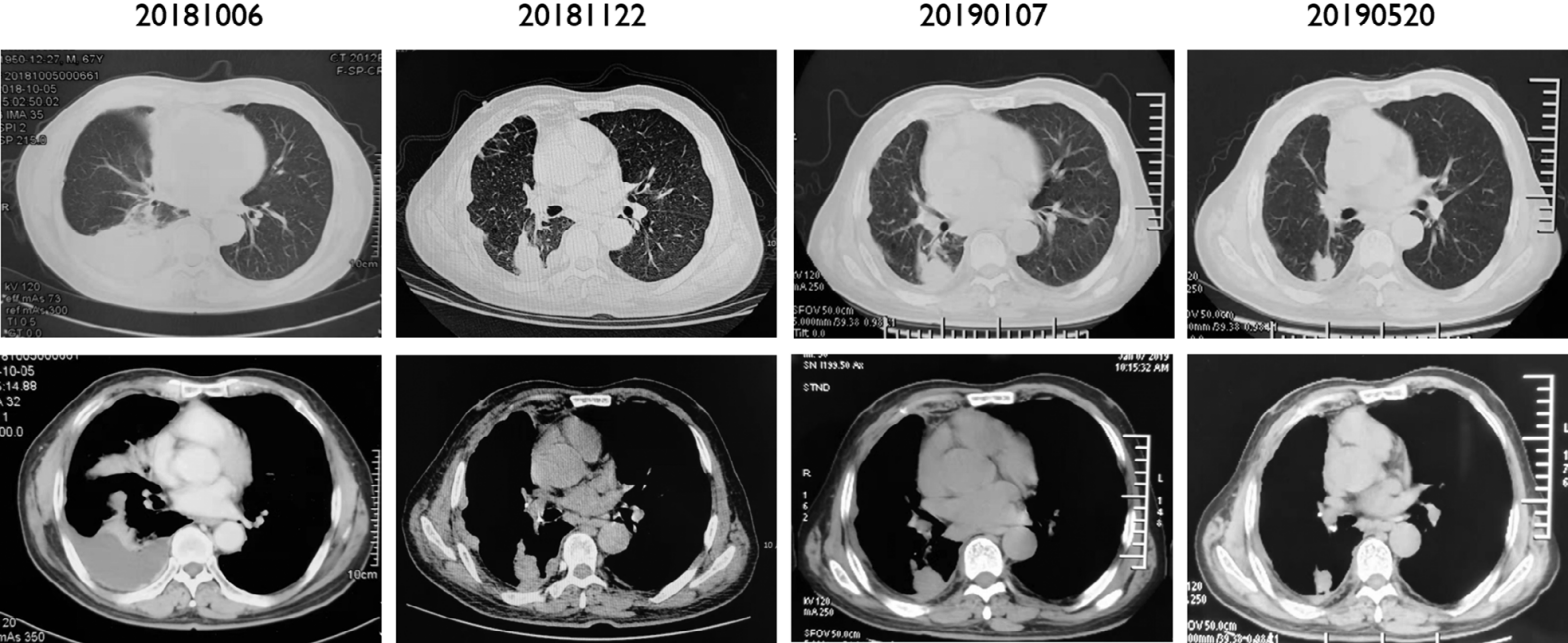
The CT scan during the treatment. “20181006” were the imaging findings before chemotherapy; “20181122” were the imaging findings after chemotherapy (before icotinib); “20190107” were the imaging findings after icotinib (before afatinib); “20190520” were the imaging findings 3 months after afatinib.
